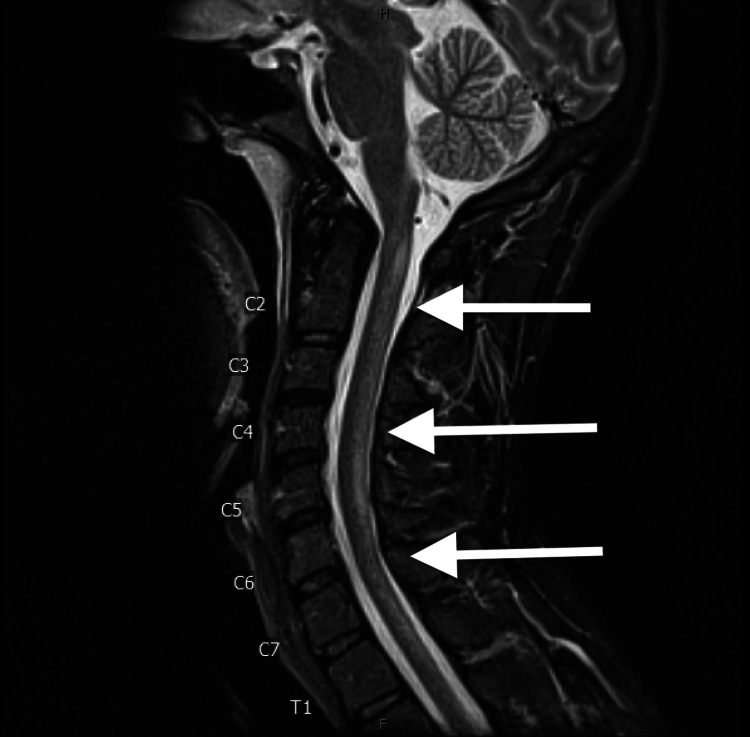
MRI of the cervical spine Moderate increased T2 signal intensity is seen from C4 to C5-C6.  Superiorly, this involves the central cord.  More caudally, it expands to the posterior cord on each side of the midline, slightly greater on the left than right.  Another cord signal abnormality is seen dorsally at C1.  Demyelination or inflammation would be favored.
